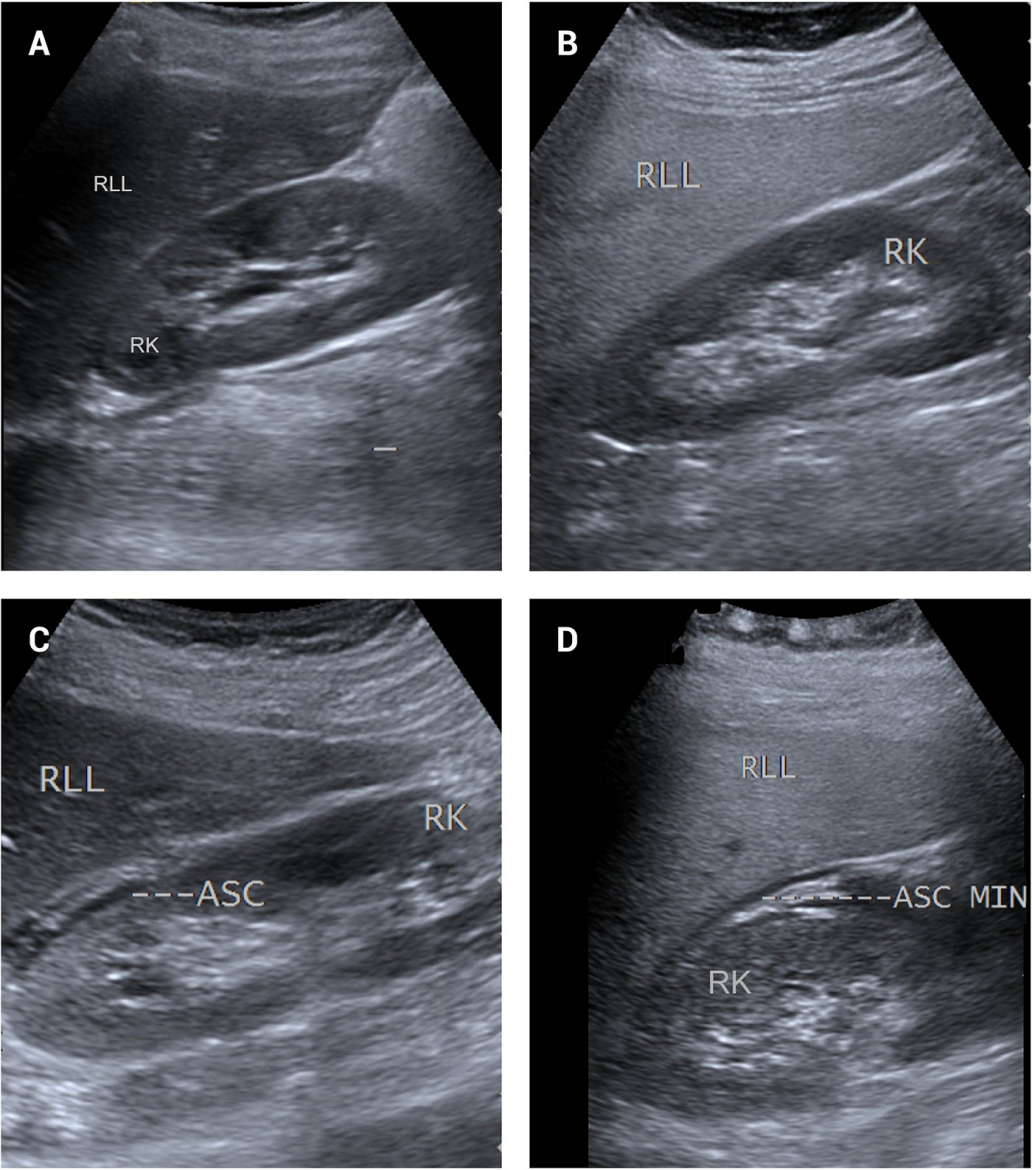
Ultrasonography findings of the four groups studied. (A) No leakage-NAFLD group: Normal liver echogenicity without pleural effusion/ascites. (B) No leakage+NAFLD group: Increased liver echogenicity relative to the renal parenchyma without pleural effusion/ascites. (C) Leakage-NAFLD group: Normal liver echogenicity with ascites. (D) Leakage+NAFLD group: Increased liver echogenicity relative to the renal parenchyma with ascites. Abbreviations: A: ascites; RK: right kidney; RLL: right liver lobe; MIN: minimal.
